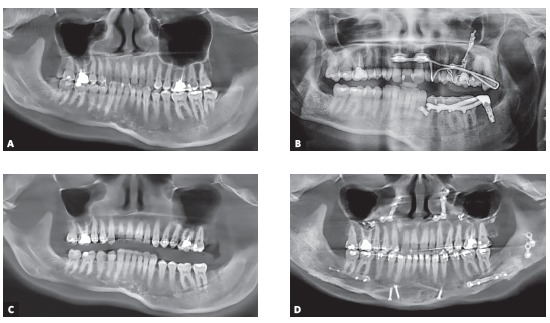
Panoramic radiographs: A) Before molar intrusion; B) During molar intrusion; C) Before orthognathic surgery; D) 10 months after orthognathic surgery.
